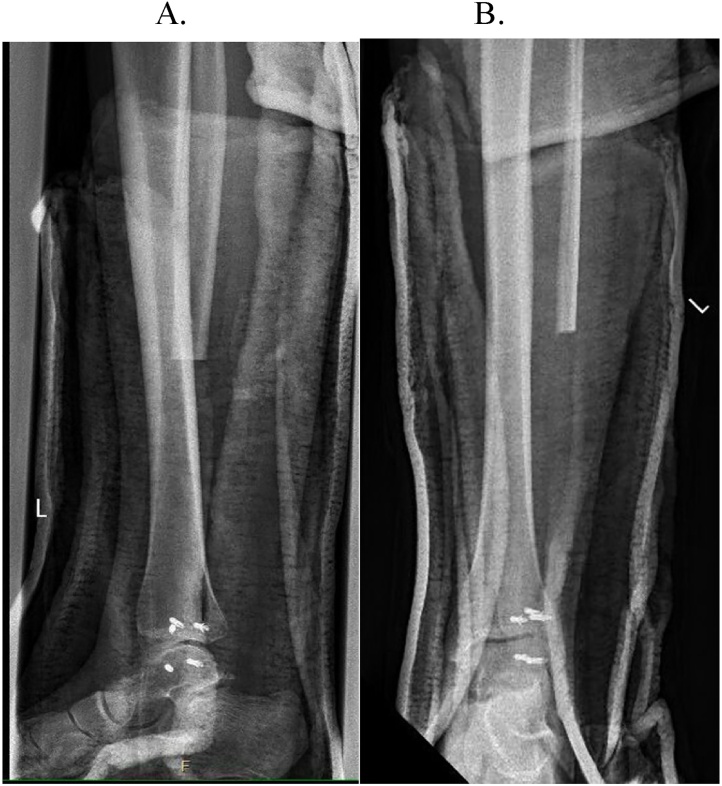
A and B) Immediate postoperative radiographs of patient #2.
